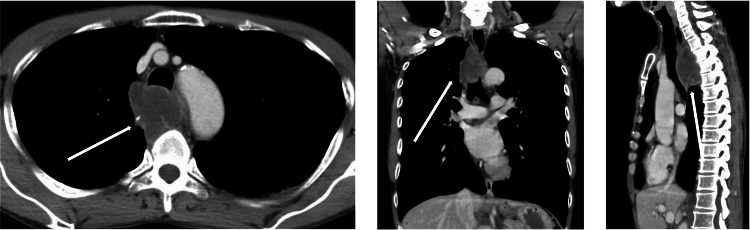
Chest computed tomography (CT) findings A mass is noted on the dorsal bronchus and right side of the esophagus before preoperative chemotherapy.
